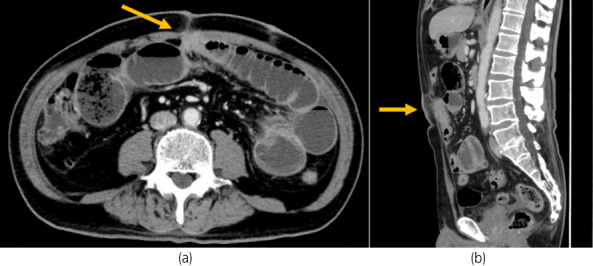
(a, b) Abdominal CT scans showed dilatation on the mouth side of the small intestine in contact with the camera port.
